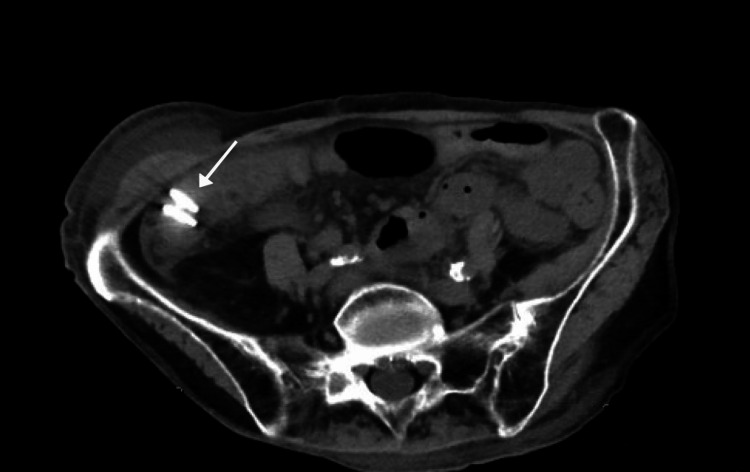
A CT scan of abdomen/pelvis. The white arrow shows the endoclip markers within the right colon and minimal surrounding inflammatory changes, which is indicative of a recent polypectomy.
